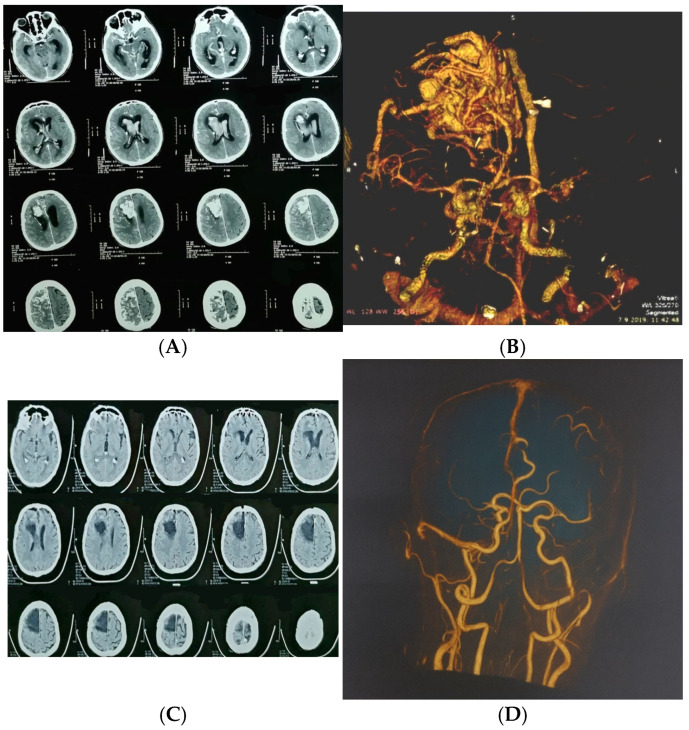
A 65-year-old man presented with severe headache, nausea, vomiting, and impaired consciousness progressing to sopor. (A) Axial CT showing large ruptured right frontal arteriovenous malformation (AVM); (B) urgent CT angiography confirmed diagnosis; (C) postoperative computed tomography; (D) postoperative computed tomography angiography.
